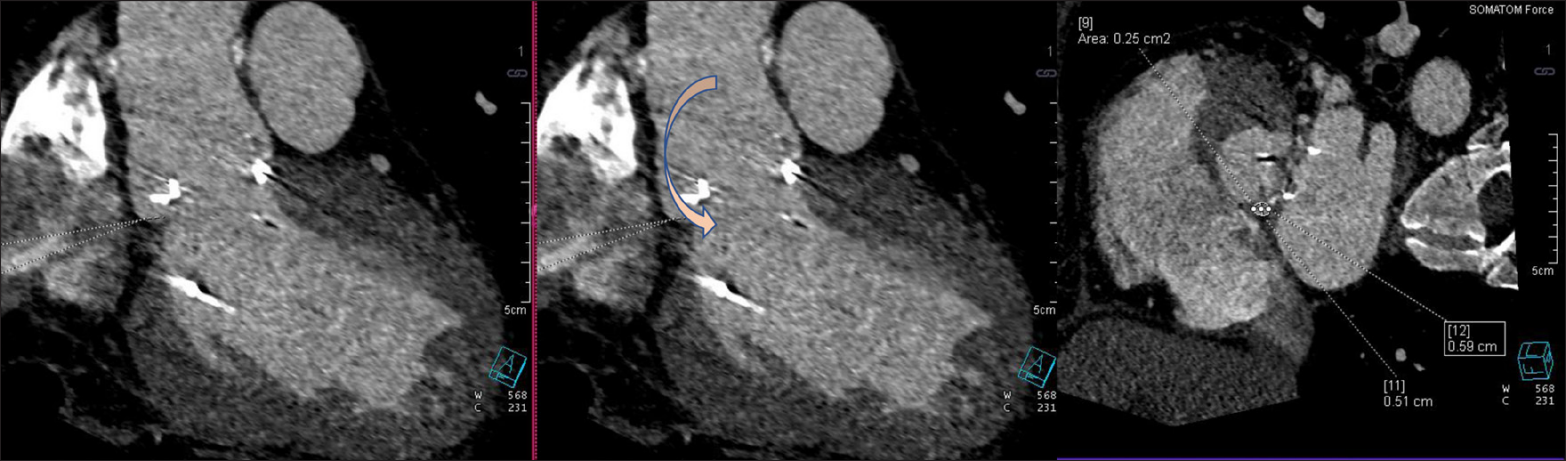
Cardiac computed tomography showing surgical aortic valve with para-valvular leak (arrow).
